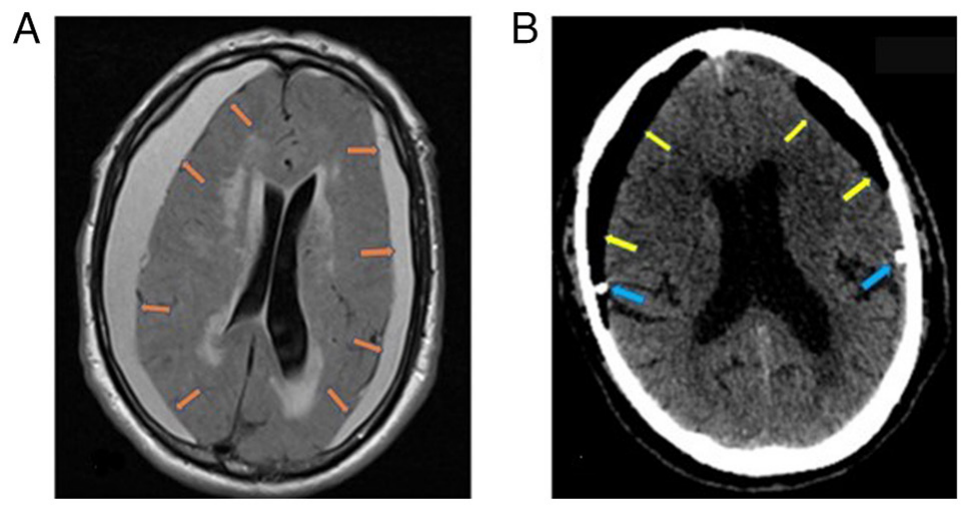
(A) A brain MRI revealed bilateral chronic subdural hematomas. The orange arrows indicate the brain-to-hematoma interface. (B) The post-operative computed tomography scan revealed that the hematoma was completely evacuated, and the cavity (yellow arrows) was filled with air as the brain failed to re-expand. The draining catheters (blue arrows), connected to a closed collection system, are in place.
